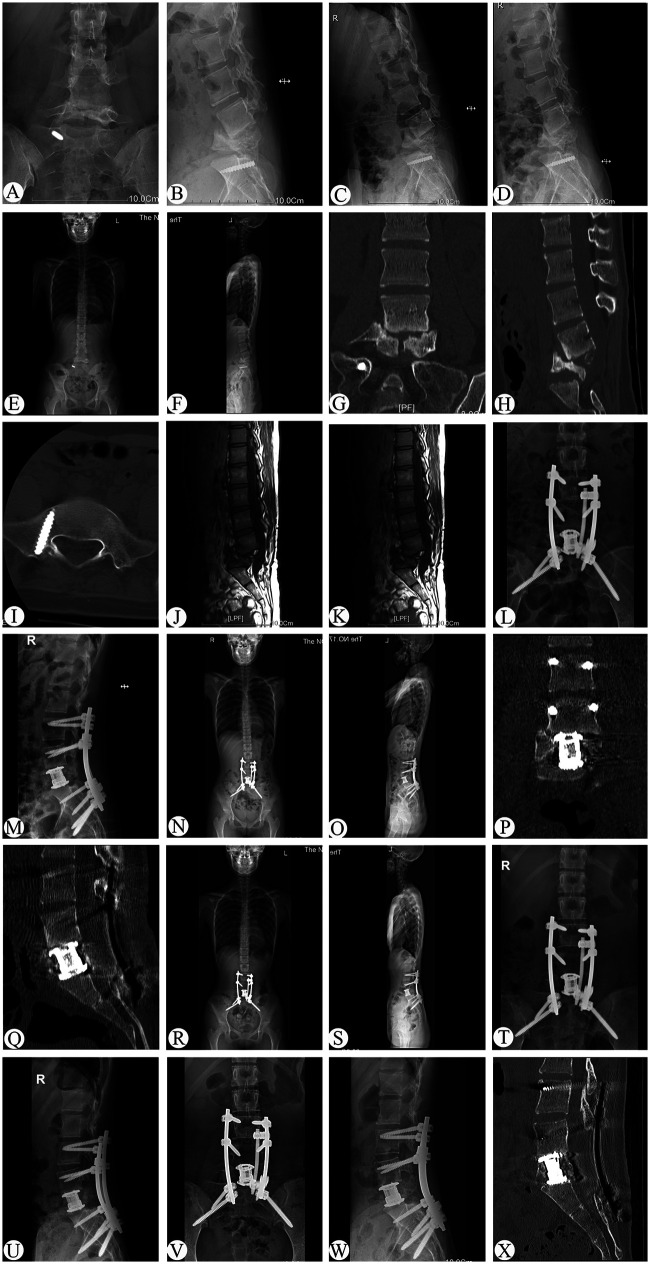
Preoperative, postoperative, and follow-up radiographs of a 49-year-old patient the patient who had dated L5 vertebral fractures with kyphotic deformity managed by anterior vertebrectomy and reconstruction with O-arm navigation (case 8). (A-K) x-ray, CT, and MRI before operation. (L–Q) x-ray and CT at 3 days after operation. (R–U) x-ray at 1 years follow-up. (V–X) x-ray and CT at 2.5 years follow-up.
